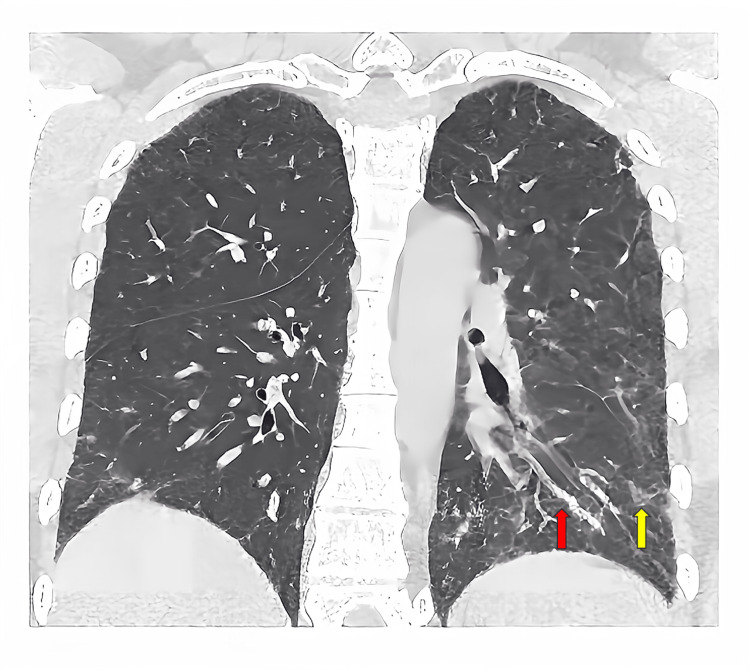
High-resolution CT of the chest showing subpleural and peribronchovascular bilateral reticulations (red arrow) predominately in lower lobes and lingula with mild ground glass opacities and traction bronchiectasis (yellow arrow) without honeycombing, cavitation, or effusions as well as enlargement of the main pulmonary artery concerning for pulmonary hypertension.
